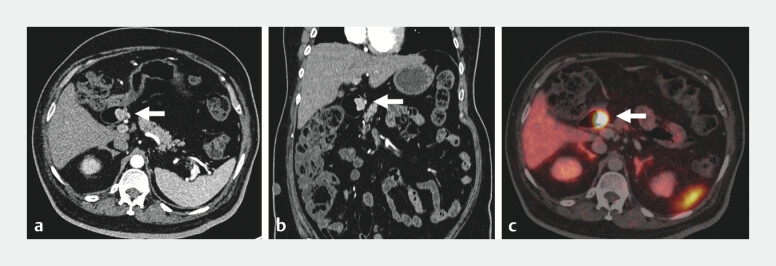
Computed tomography (CT) imaging.
